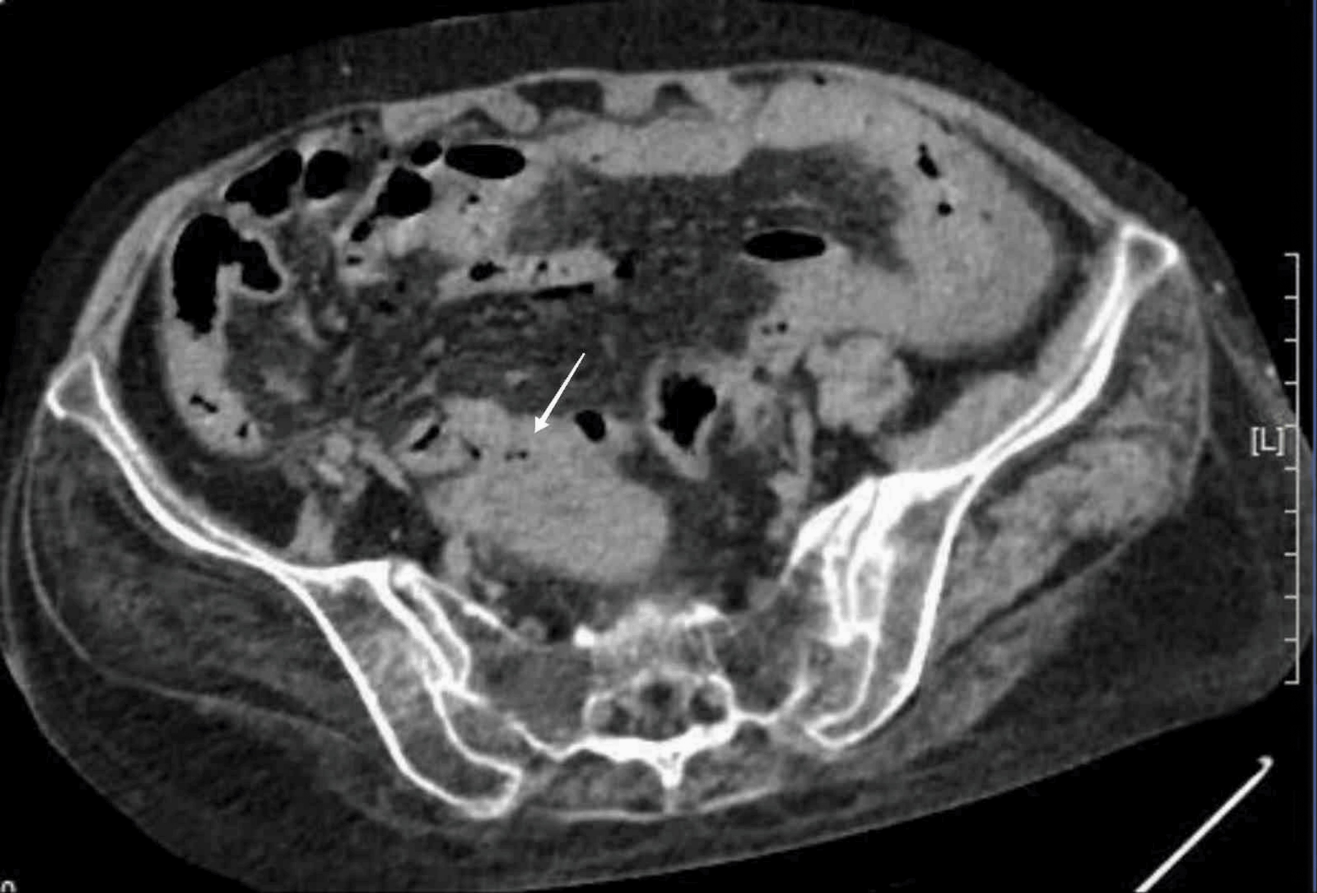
CT scan of the lower abdomen shows mixed-density shadow at the fundus of the uterus
